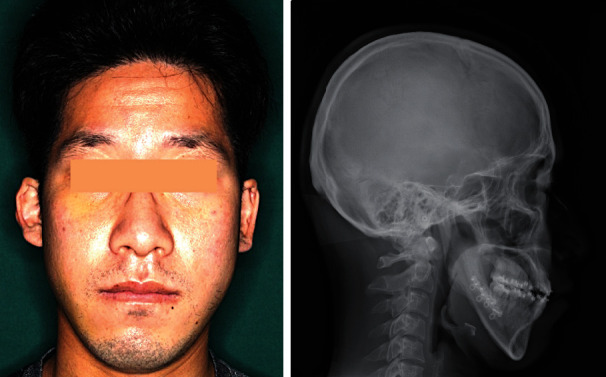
Postoperative facial photograph and lateral cephalometric radiograph. Mandibular deviation and occlusal relationships are improved.
